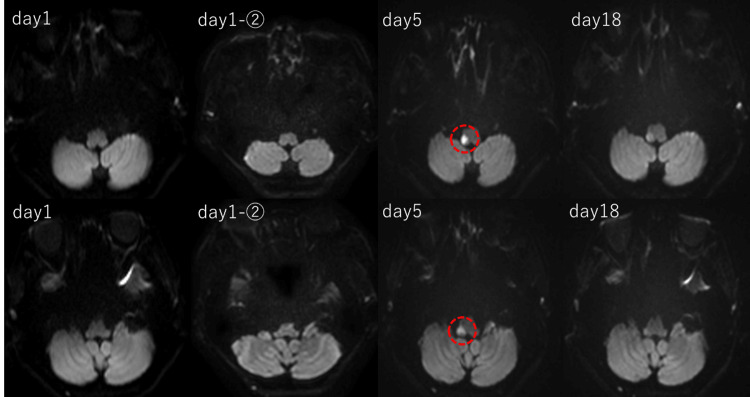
The temporal changes are shown with diffusion-weighted imaging (DWI) (B-value = 1000 s/mm²). The infarct in the right lateral medulla was not visualized on the initial two magnetic resonance imaging (MRI) scans and was first identified on MRI performed on day 5 after onset. MRI findings suggest that the branches of the right posterior inferior cerebellar artery (PICA) supplying the cerebellar hemisphere were preserved, while those supplying the medulla were involved.
